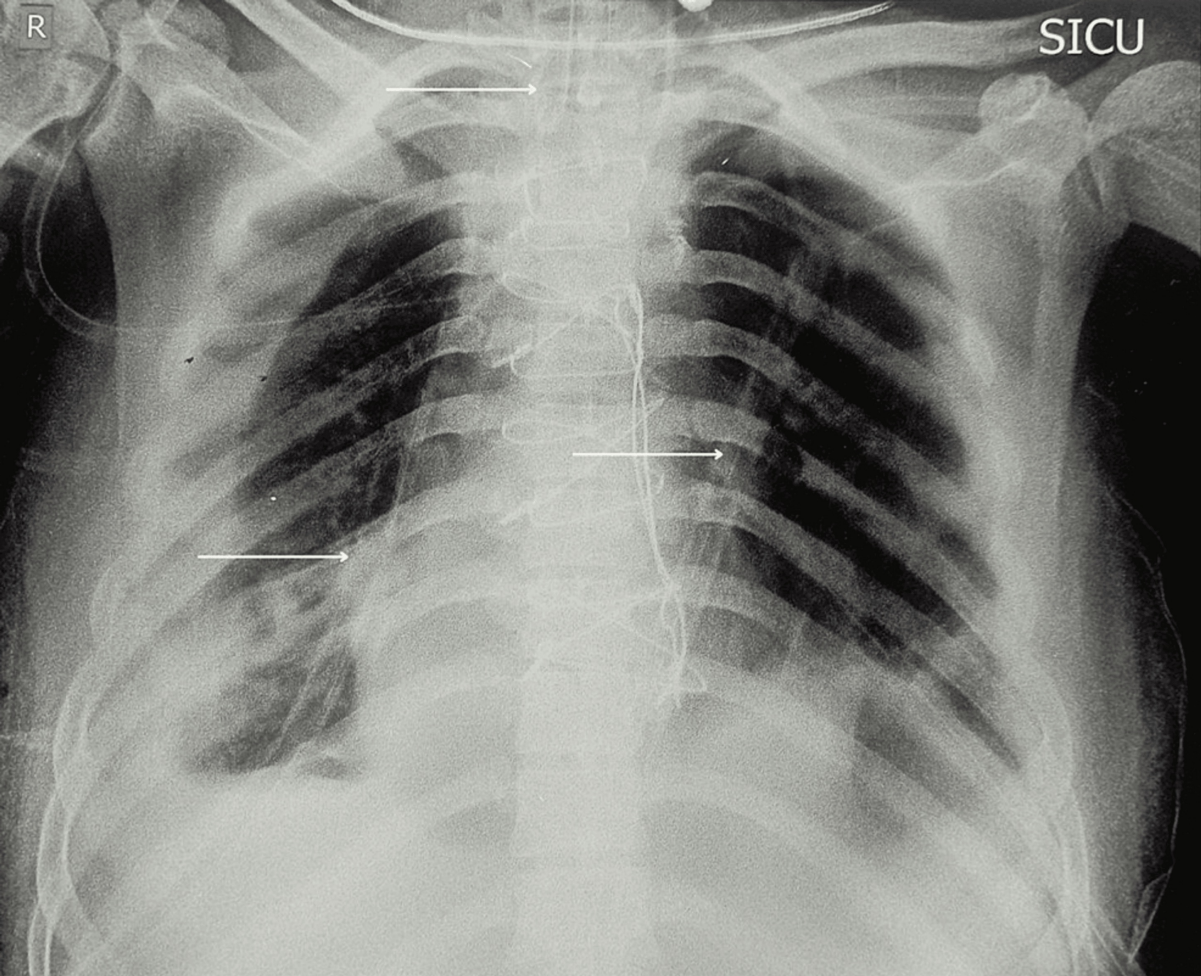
Postoperative chest X-ray showing bilateral pneumothorax and multiple intercostal drains (white arrows) SICU: surgical intensive care unit
